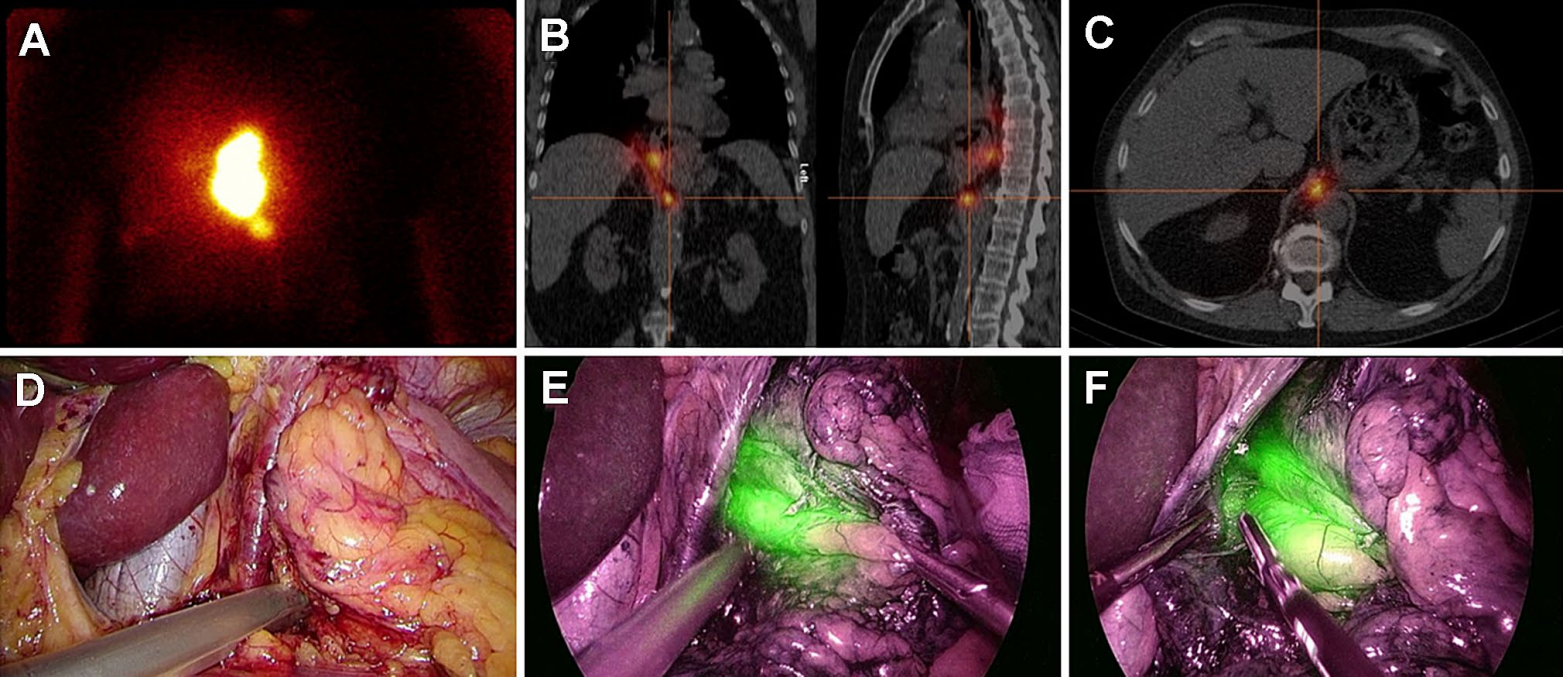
Identification of a retrocrural located sentinel node. A: Lymphoscintigraphy 120 min after injection of the hybrid tracer showed the injection site and a sentinel node located below. B + C: This was combined with a SPECT/CT of the thorax and abdomen to detect the exact sentinel node location. D: High radioactivity uptake was confirmed with the laparoscopic gammaprobe during the abdominal phase of surgery. E: The sentinel node was also clearly visualized as indocyanine green positive after switching the camera view to near-infrared. F: Laparoscopic resection of the sentinel node was started while visualized with the near-infrared camera
